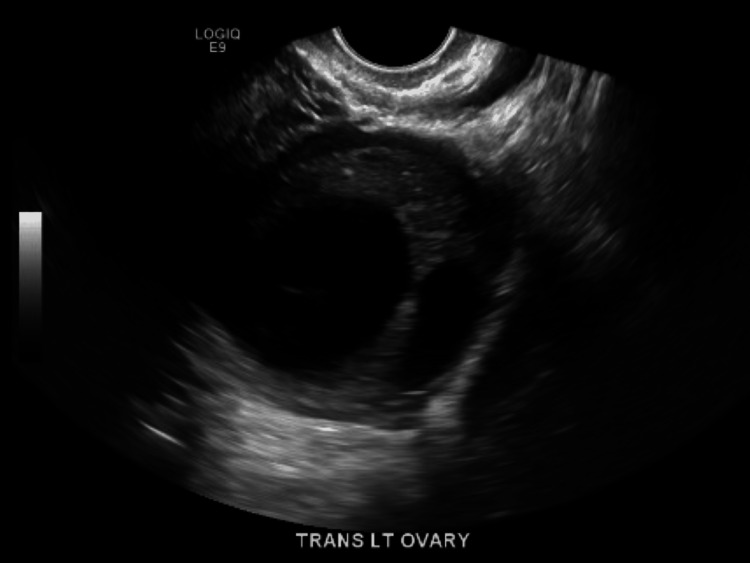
Ultrasound transvaginal view of the left ovary This is another view of the left ovary, showing the size of the left ovary, cystic focus, and left ovarian lesion.
